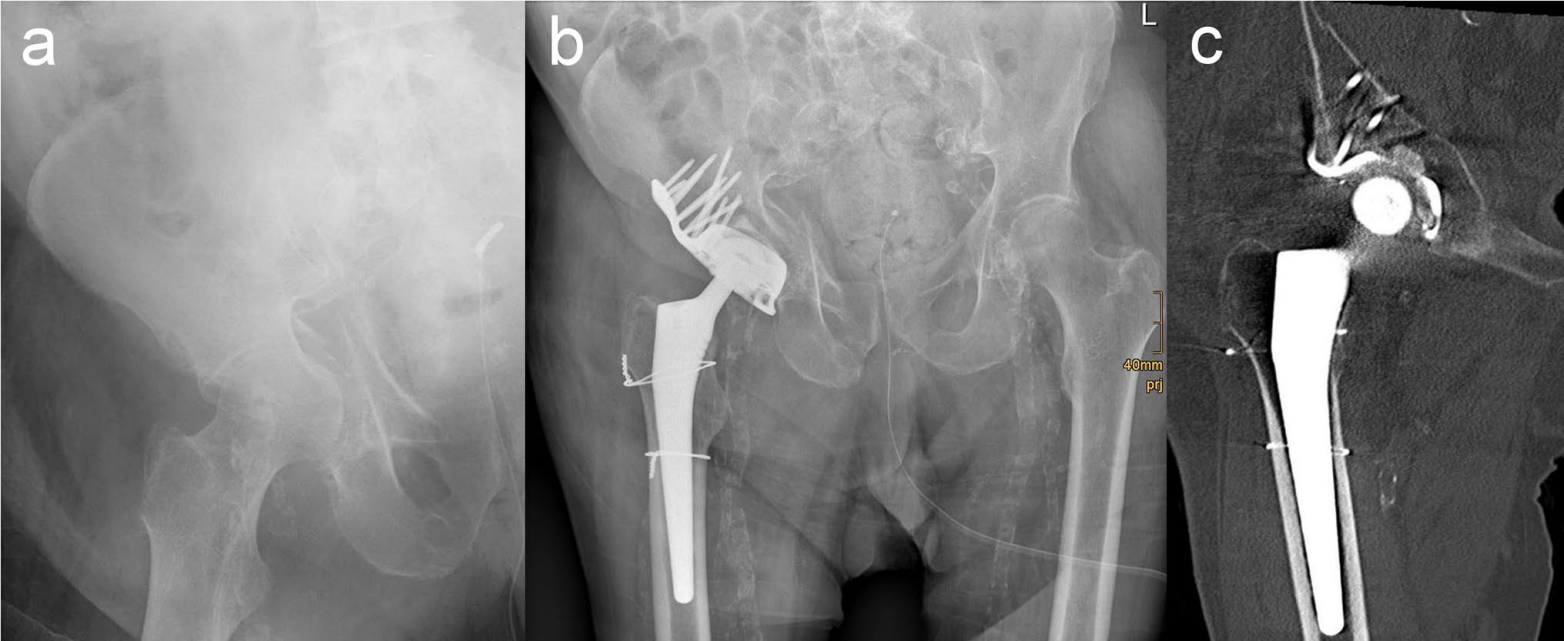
a X-ray of a displaced ACPHT fracture. b Ap view of the hip joint with ARRP and THA 6 months after surgery. c CT imaging confirmed bony healing and osseous incorporation of the ARRP. Uneventful healing of the iatrogenic femoral fracture
